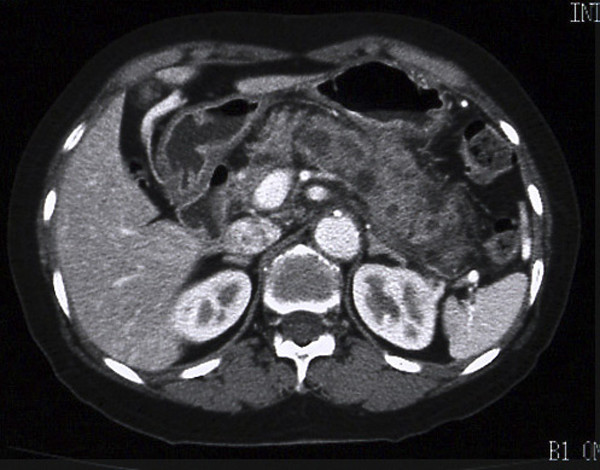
Abdominal CT just prior to surgery showing distinct swelling of the pancreas and the gastric wall, causing the border between the two organs to be unclear.
